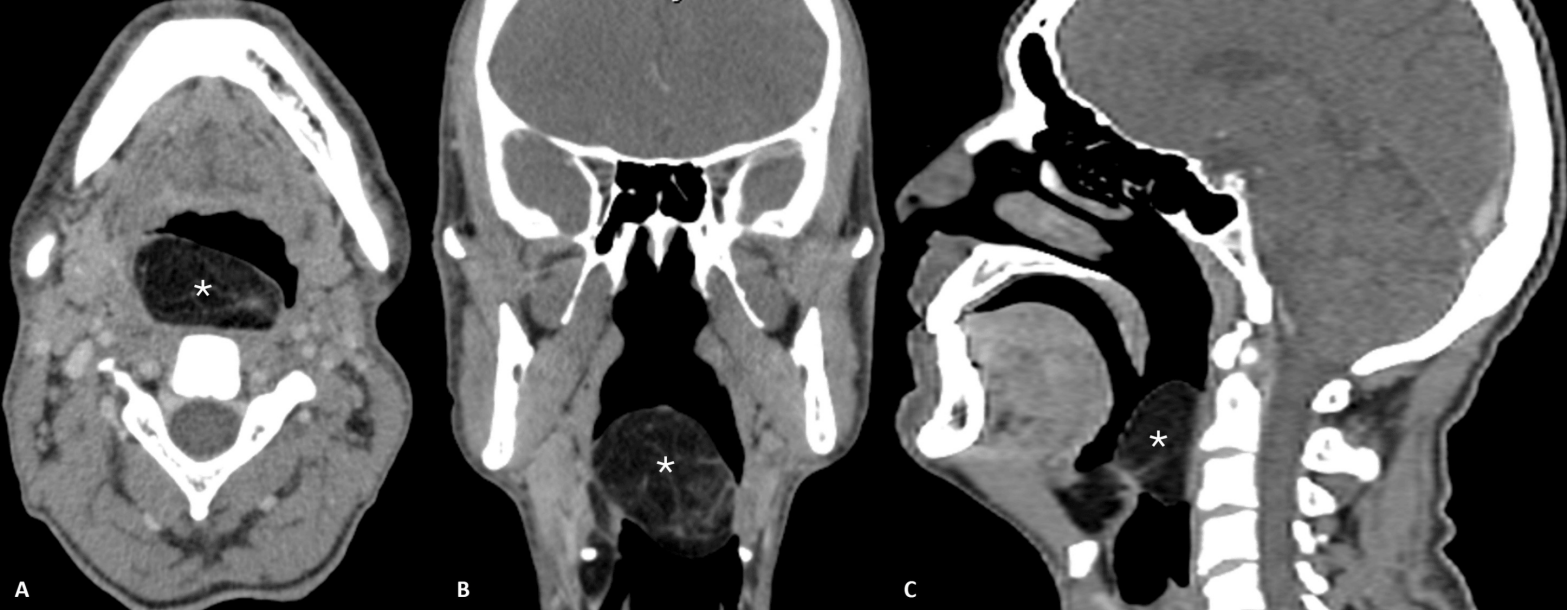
Contrast CT scan Computed tomography in transversal (A), coronal (B), and sagittal (C) views, performed at the time of diagnosis, demonstrates a low-density lesion with an apparent origin in the paraglotic space, which crosses the midline and extends to the oropharynx, obstructing the upper aerodigestive tract (asterisk).
